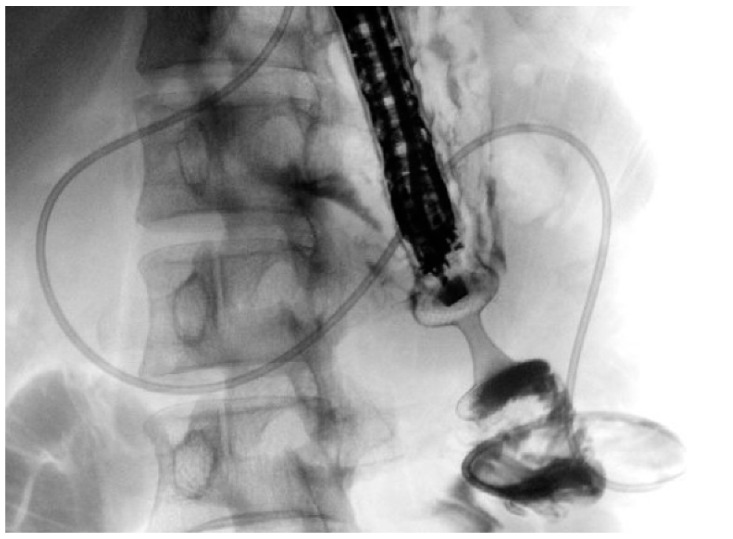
Endoscopic ultrasound-guided gastroenterostomy (EUS-GE). Fluoroscopic view of EUS-GE with contrast medium flow through the Lumen-Apposing Metal Stent (LAMS) lumen from the jejunum to the stomach. The copyright of the image belongs to the authors.
